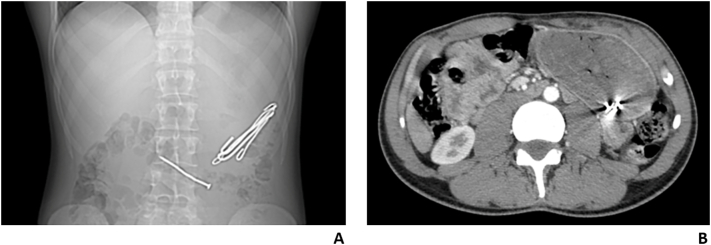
Radiological findings. A: plane radiography B: CT scan.
